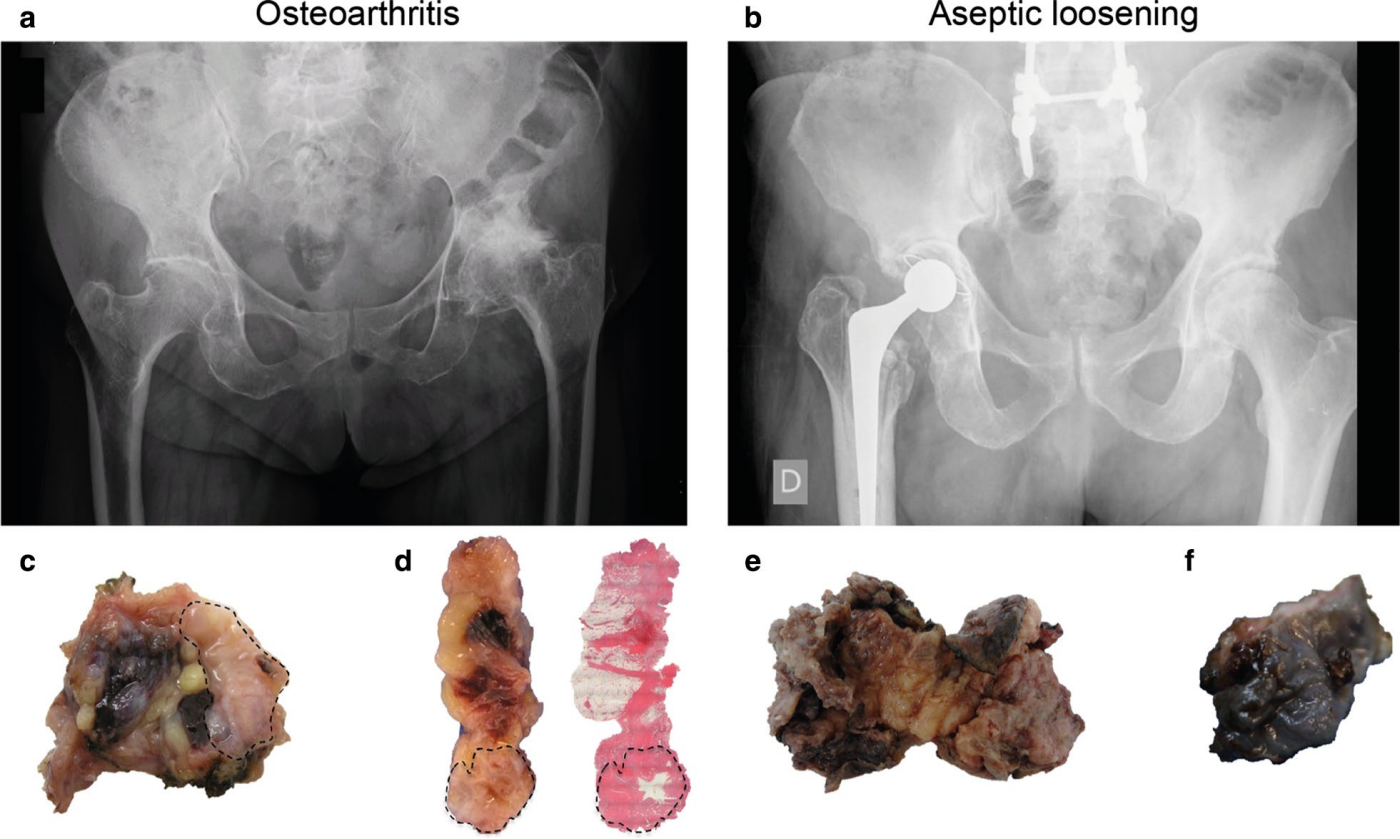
Radiological and macroscopic tissue features of OA, AL and metallosis. a Anteroposterior X-rays showing OA of the hip. b Failed metal-on-polyethylene total hip joint due to AL. c, d Macroscopic images of OA synovial tissue collected at primary hip replacement. e Synovial membrane-like interface tissue retrieved at hip revision surgery due to AL. f Metallic debris accumulation in synovial membrane-like tissue from AL patient with metallosis
